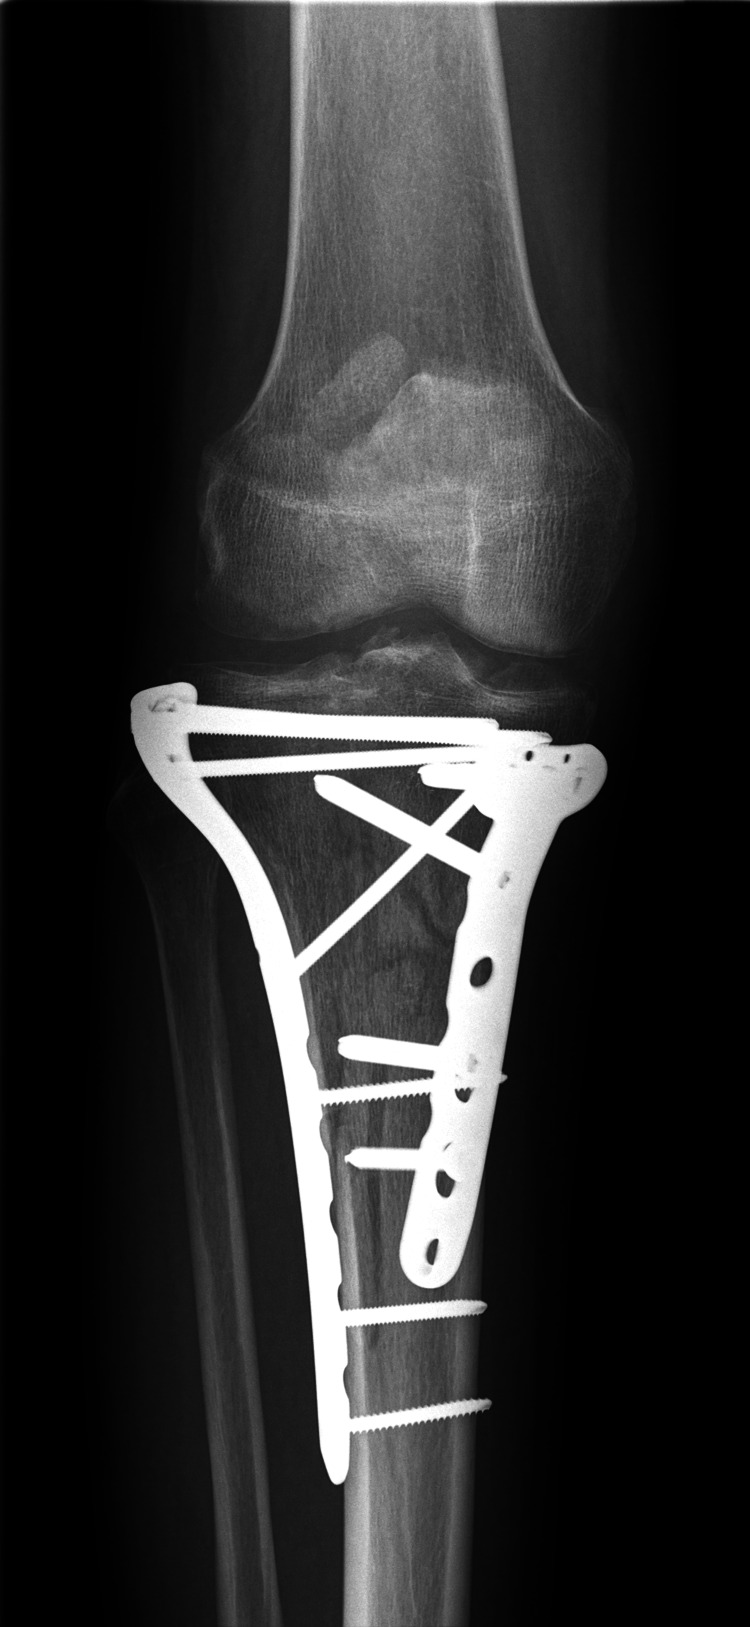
X-ray (AP view) showing the fracture after surgical intervention
